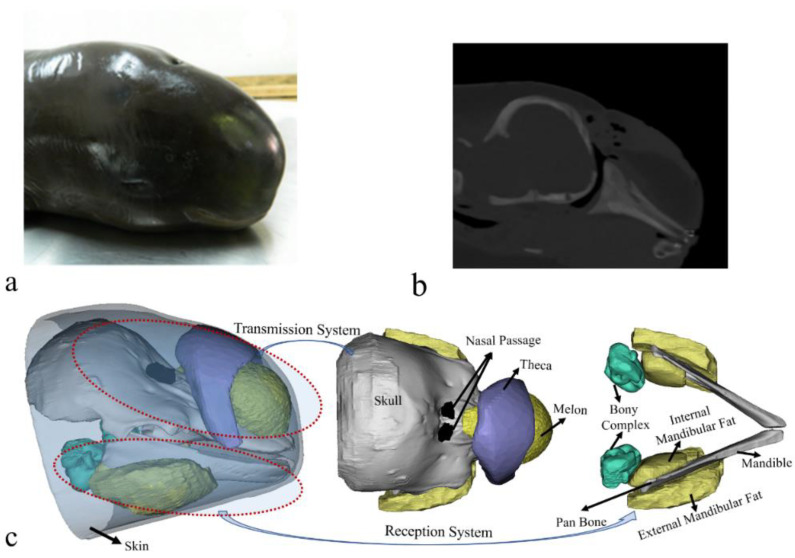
(a) A photo of the finless porpoise. (b) A computed tomography scanning image of the porpoise head in a sagittal cross-section. (c) Reconstruction of the finless porpoise showing the forehead transmission system and reception system in the lower head. The transmission system in the forehead includes the cranium, nasal passage, theca, melon, and upper jaw components. The reception system in the mandibular region consists of ear bones (bony ear complex), internal mandibular fat, external mandibular fat, and mandible.
